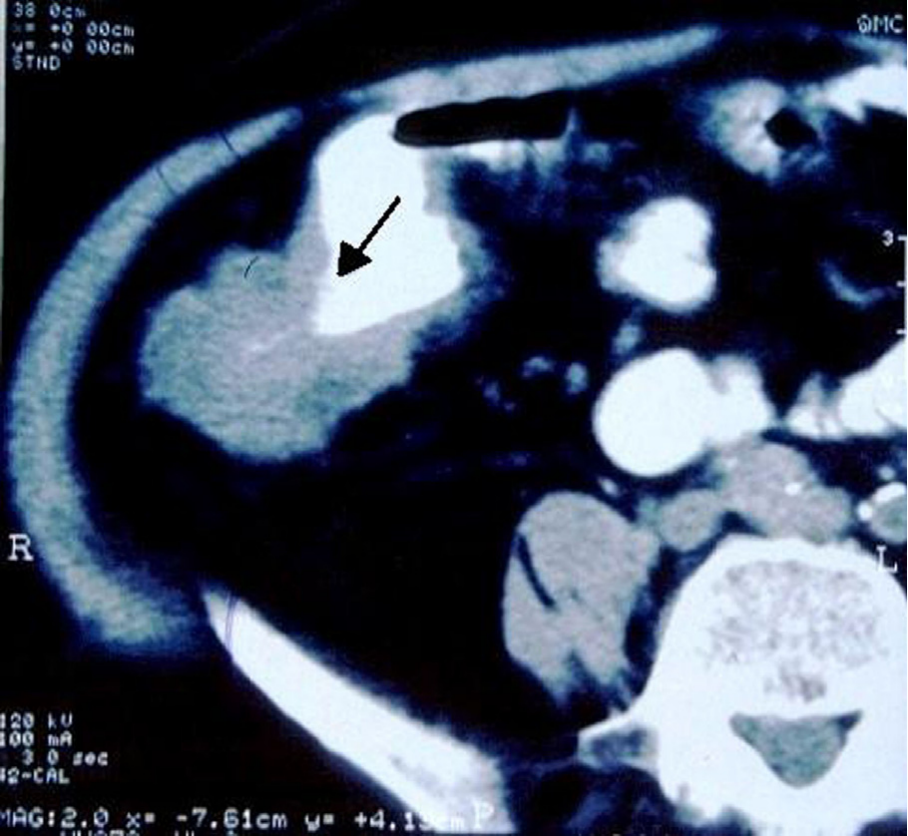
Abdominal computed tomography revealing the site of the obstruction.
